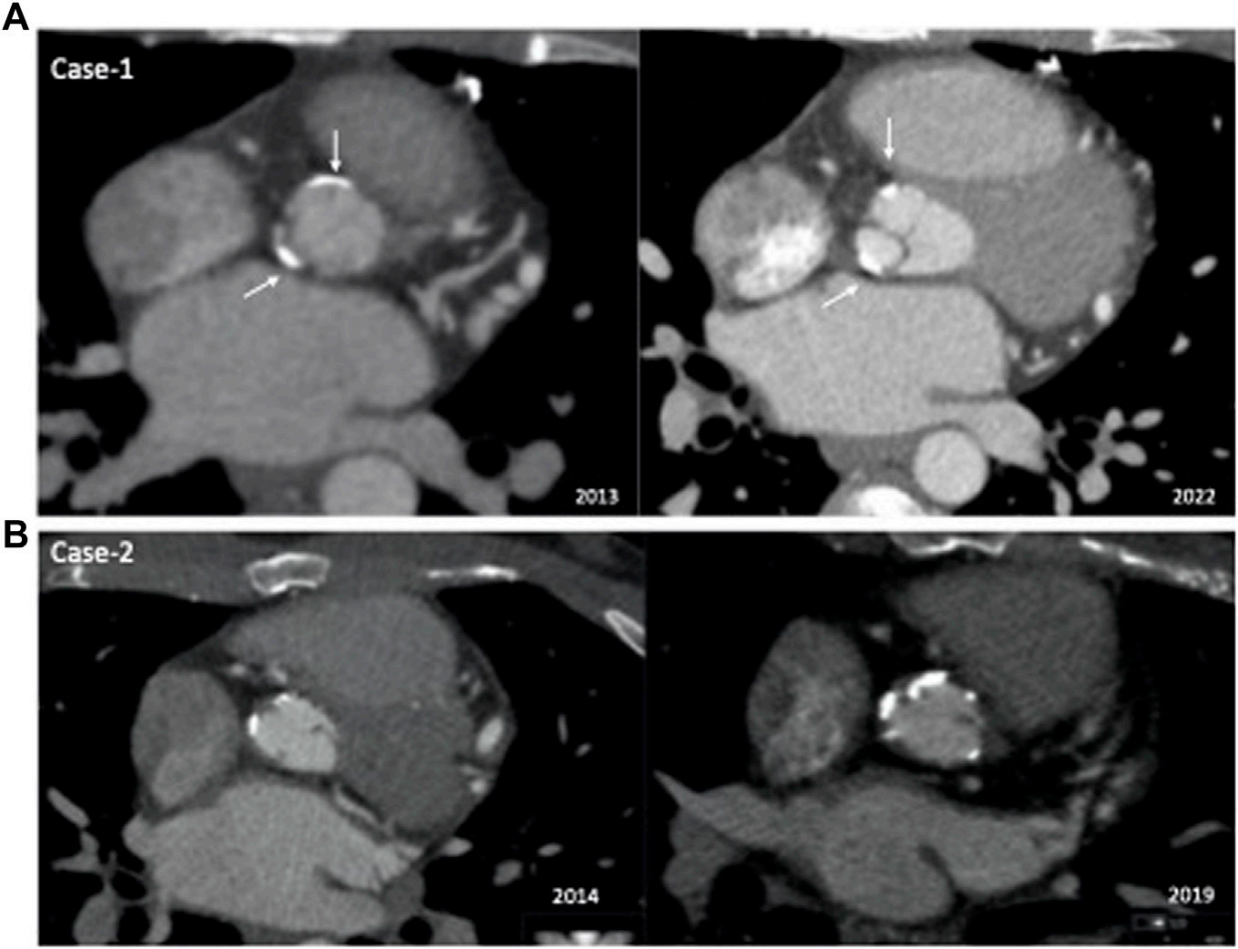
Comparison of baseline and follow-up computed tomography angiography images of the patients. (A) Case 1: Aortal involvement was almost identical between the baseline (2013) and follow-up (2022) images. (B) Case 2: Comparison of 2014 and 2019^−ΔΔCT^ angiograms showed significant progression of calcifications in the aortic root.
